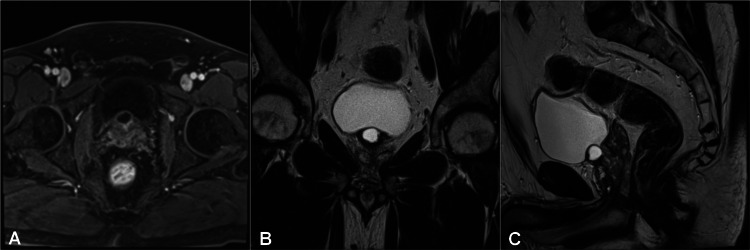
Pelvic MRI image showing an anteriorly positioned midline prostatic cyst projecting at the bladder neck region with no evidence of communication with the urethra. (A) Axial T1-weigthed MRI image. (B) Coronal and (C) Sagittal T2-weighted MRI images.
